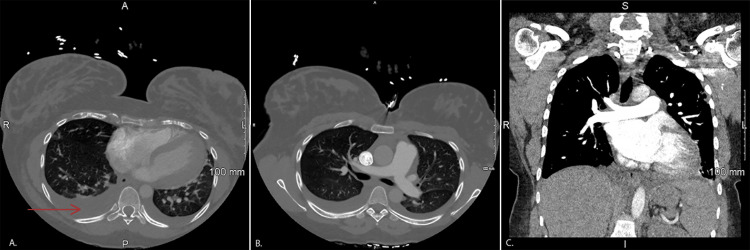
CT chest demonstrating mild bilateral effusions and negative pulmonary embolism A. Lower lung field plain with an arrow indicating pleural effusion present bilaterally. R: Right, L: left, A: Anterior, P: Posterior B. Thoracic CT with clear main pulmonary artery indicating negative saddle embolus. C. Coronal CT angiogram chest example of clear pulmonary arteries indicating negative pulmonary embolism. S: Superior; I: Inferior
